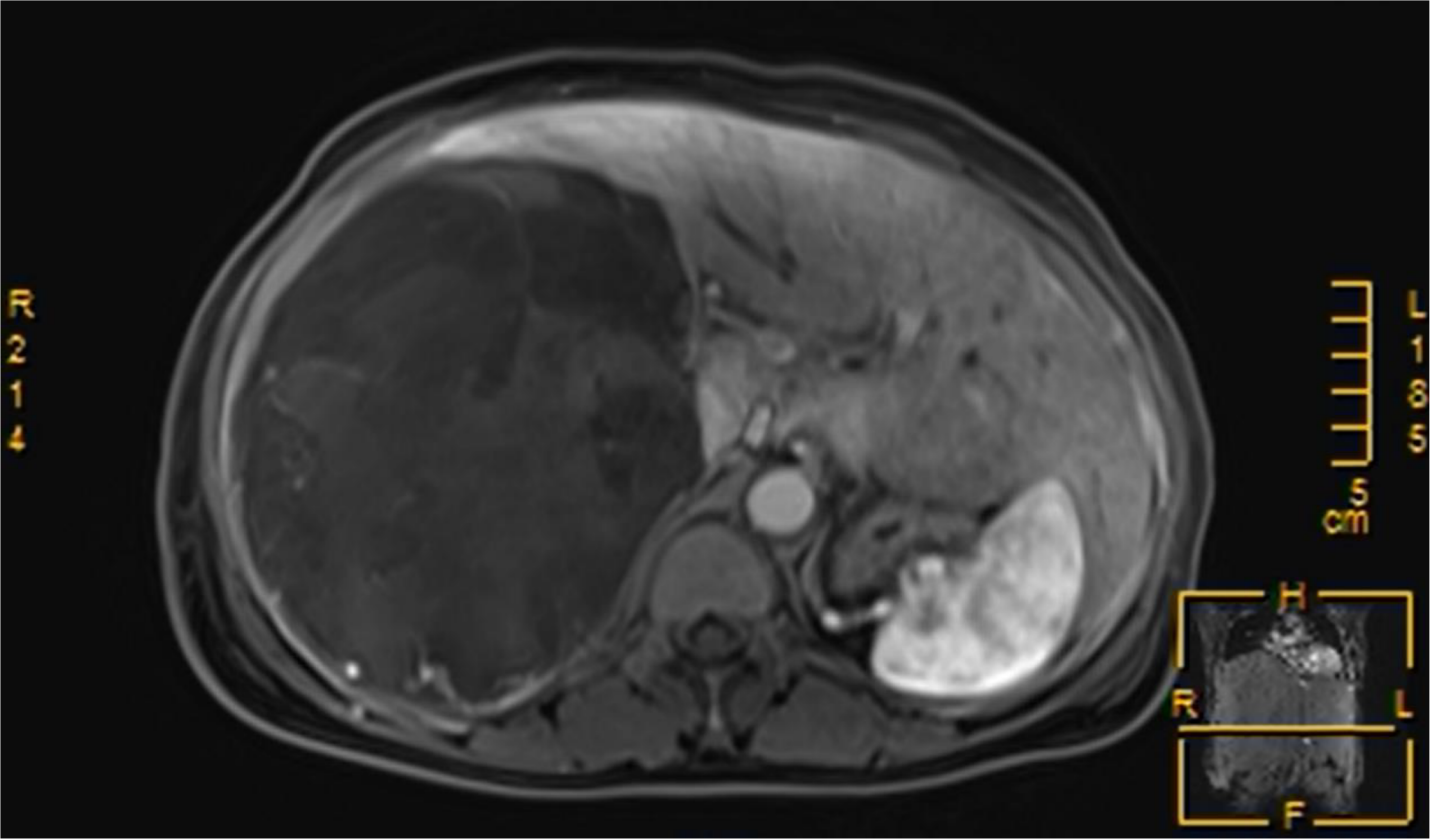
Contrast-enhanced magnetic resonance imaging demonstrated multiple patchy and linear areas of enhancement within the lesions.
